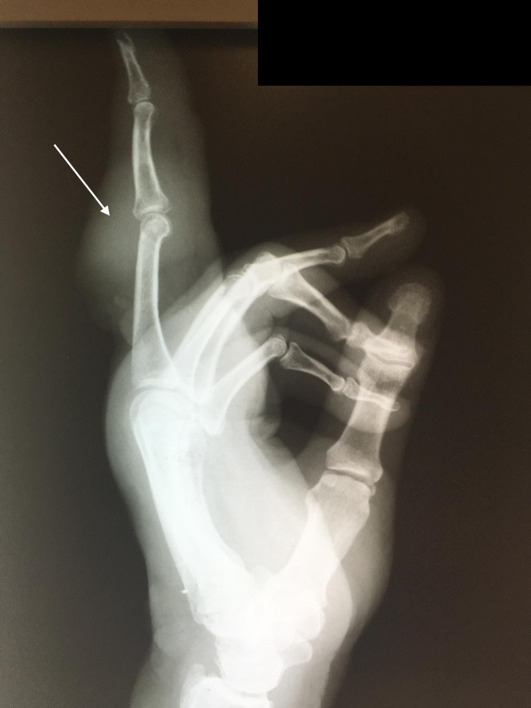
Lateral radiograph of the left hand revealing the radiopaque lesion on the dorsal aspect of the third digit, centered at the PIP joint.
